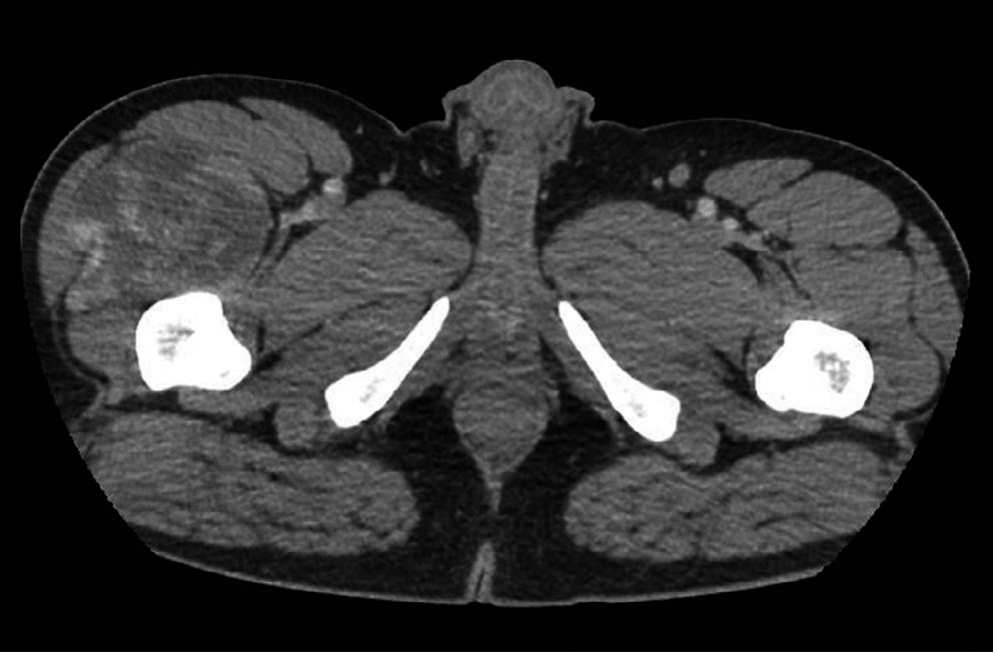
CT Abdomen/pelvis demonstrating 7.3 × 8.3 cm soft tissue mass with mass effect
